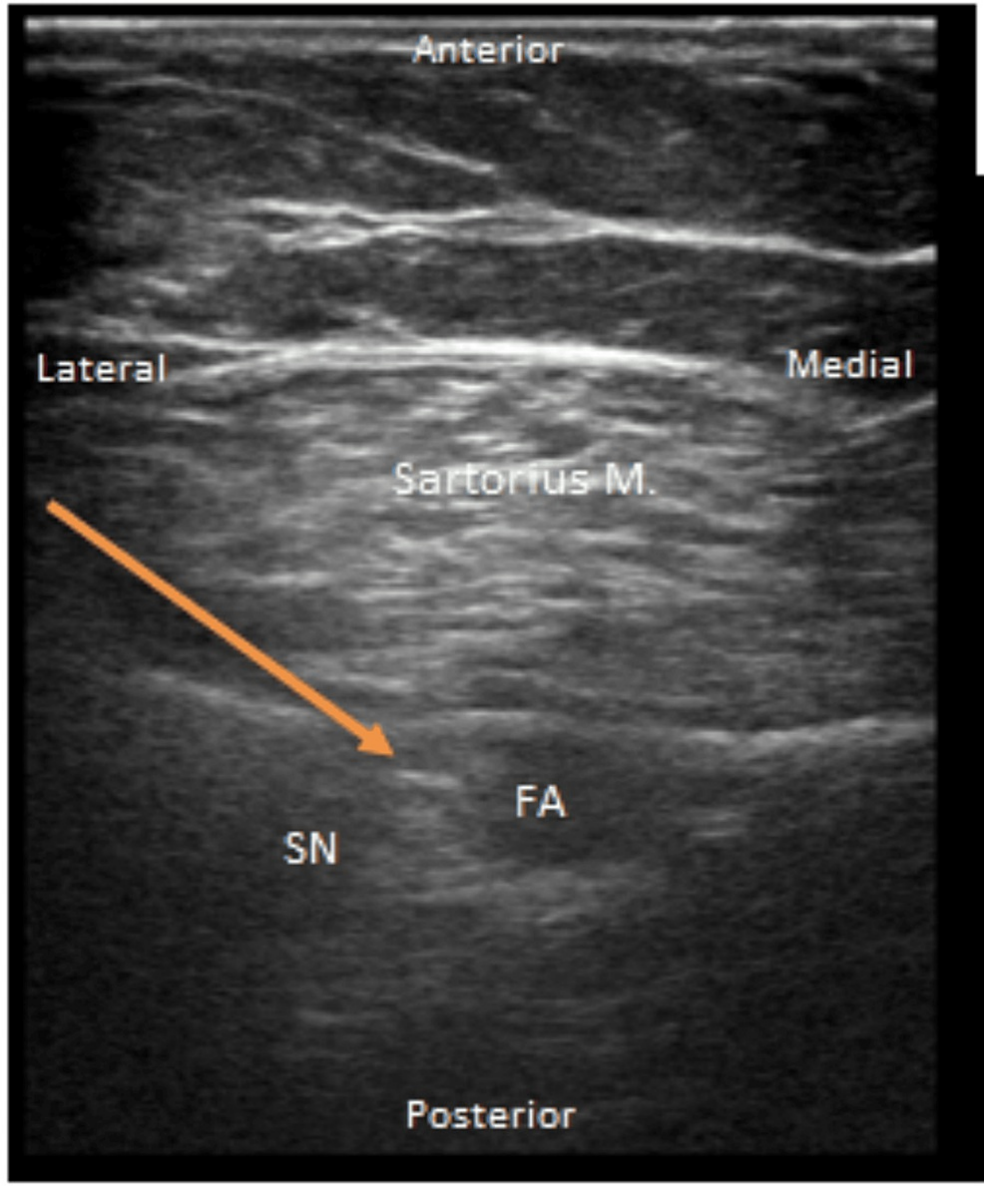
Ultrasound-guided right adductor canal catheter placement with linear probe The catheter was at a skin depth of 10 cm and the needle was inserted at a depth of 6 cm. Tuohy 18 g 10 cm needles with 20 g catheters and injection assessment showed negative aspiration, and no paresthesia on injection. Orange arrow: the in-plane tip of the needle SN: saphenous nerve; FA: femoral artery
